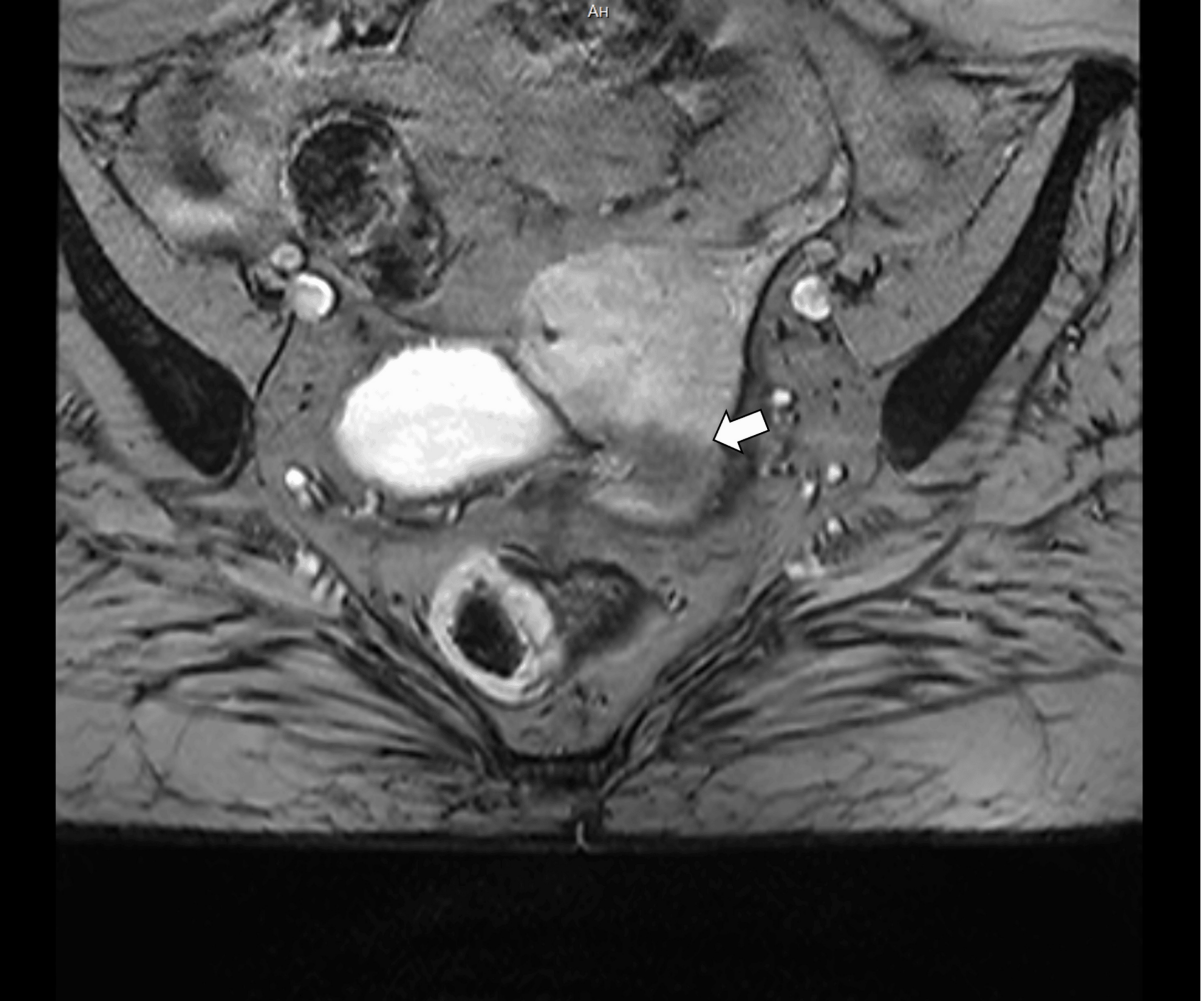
T2-weighted gradient echo (GRE) TRA image showing a heterogeneous mass with areas of intrinsic signal variation (arrows), suggestive of hemorrhagic or necrotic components, with arrows also indicating myometrial invasion. TRA – Transverse (axial) plane
